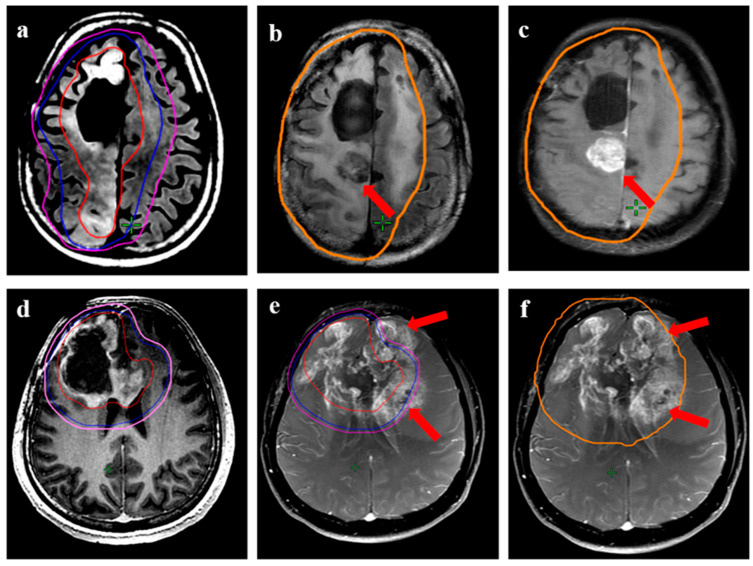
Two patients with central recurrence, each in the upper and lower panel. (a) (37-year male with IDH-mutant astrocytoma) shows target volumes (GTV: red, CTV: blue; PTV: magenta) on T2-FLAIR MRI. The CTV was drawn to include all areas of T2w hyperintensity. (b,c) is T2-FLAIR and T1w contrast images showing recurrent disease (arrow) and its relation with the 95% isodose line (orange line). (d) (56-year male with recurrent glioblastoma) shows the target volumes for another patient volumes (GTV: red, CTV: blue; PTV: magenta) on T1w contrast MRI. The GTV was the enhancing disease, with CTV drawn as an expansion of 10 mm around the enhancing disease. (e,f) show the recurrence volumes with respect to target volumes and the 95% isodose line (orange), respectively. The major portion of recurrent disease can be seen lying within the 95% isodose line.
